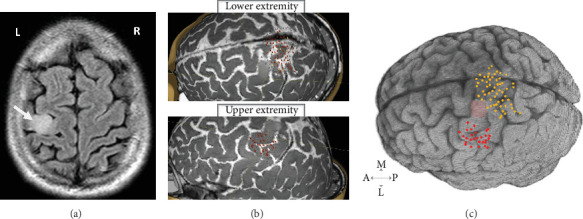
(a) Representative fluid-attenuated inversion recovery (FLAIR) MRI on admission in axial view, showing left posterior frontal lobe mass measuring 1.7 × 1.4 cm at the left precentral gyrus. L, left; R, right. (b) Preoperative nTMS-positive sites on the upper and lower extremities of the sensorimotor area superimposed on the T2-weighted MRI. Red dots represent the upper extremity and lower extremity motor-evoked potentials. (c) Coregistered nTMS points on the 3D rendering of the patient's brain. Red dots correspond to the upper extremity, and yellow dots correspond to the lower extremity. The pink shaded region shows tumor infiltration. A, anterior; P, posterior; M, medial; L, lateral.
